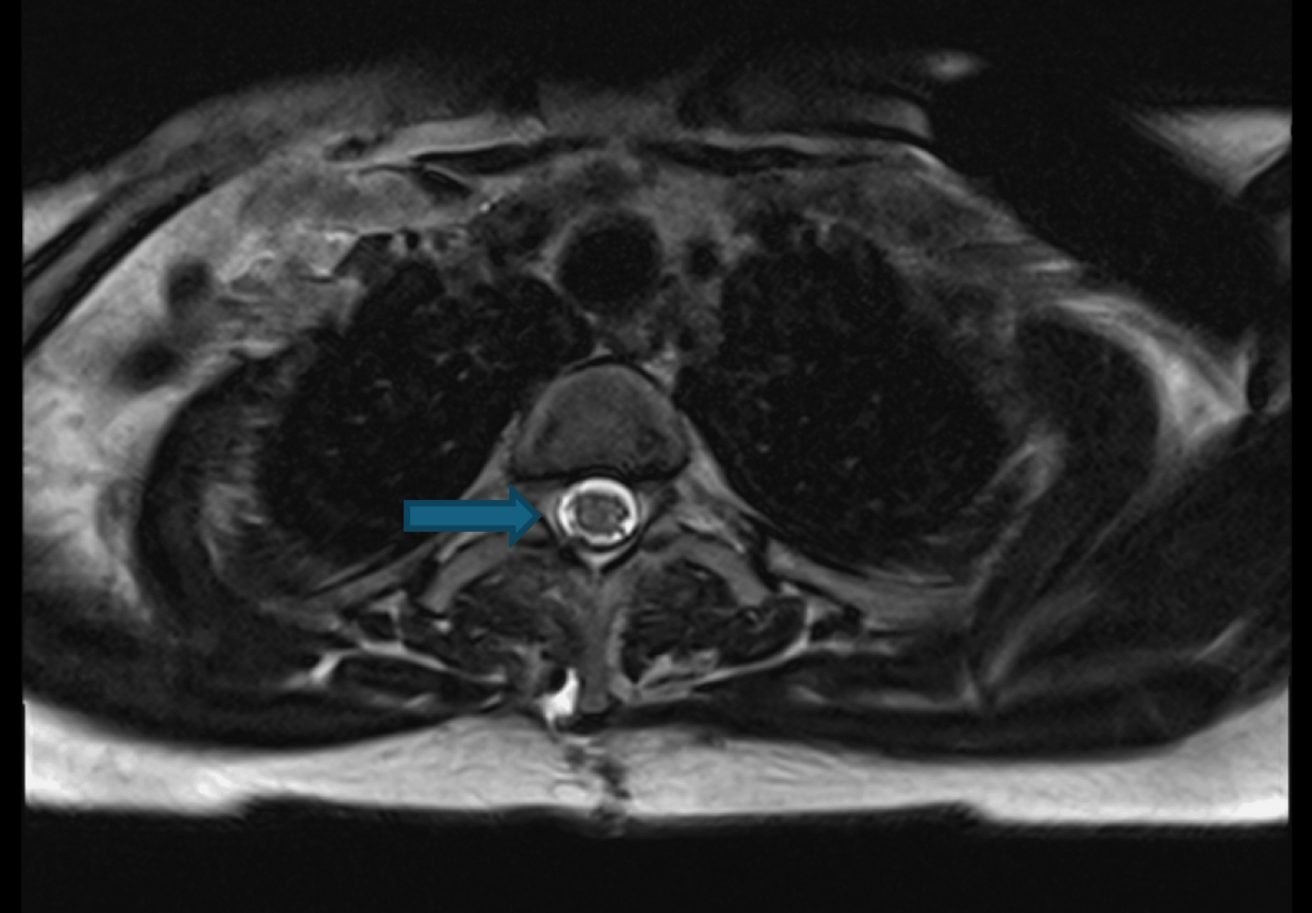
Axial T2-weighted MRI showing the post-operative resolution of intrathecal haematoma at level T3-T4. The blue arrow indicates the location of the resolved intrathecal haematoma on axial MRI.
